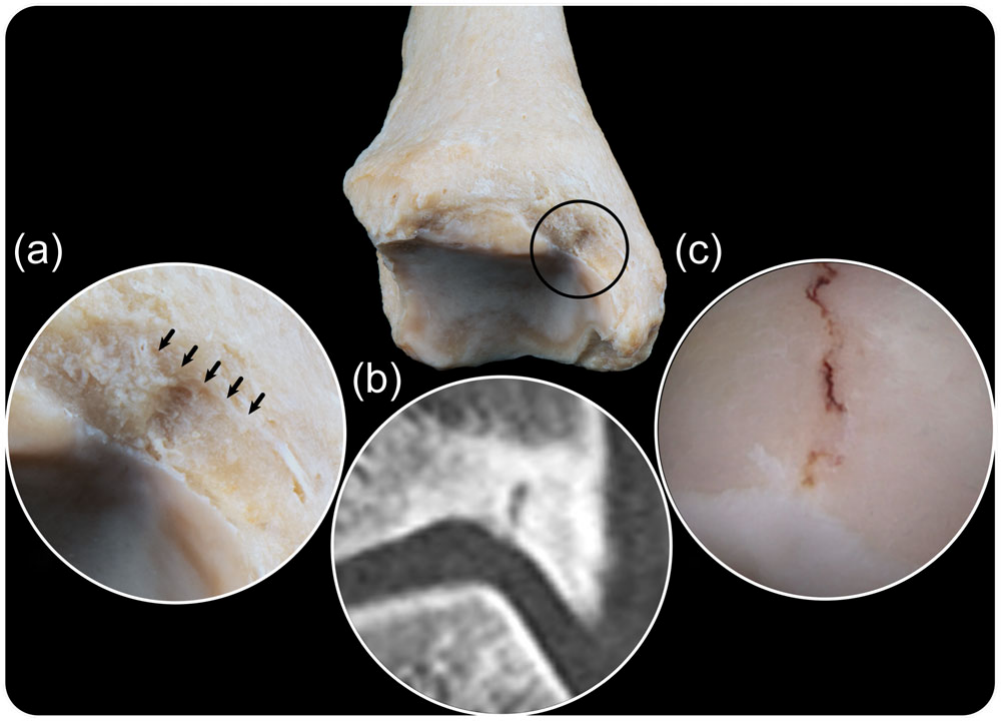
Image showing the anatomy of the distal tibia, including the medial malleolus and Harty's notch. (a) Magnification of the bony anatomy with Harty's notch highlighted (black arrows). (b) Computed tomography scan of a patient with a medial malleolus stress fracture. (c) Arthroscopic image of a medial malleolus stress fracture.
